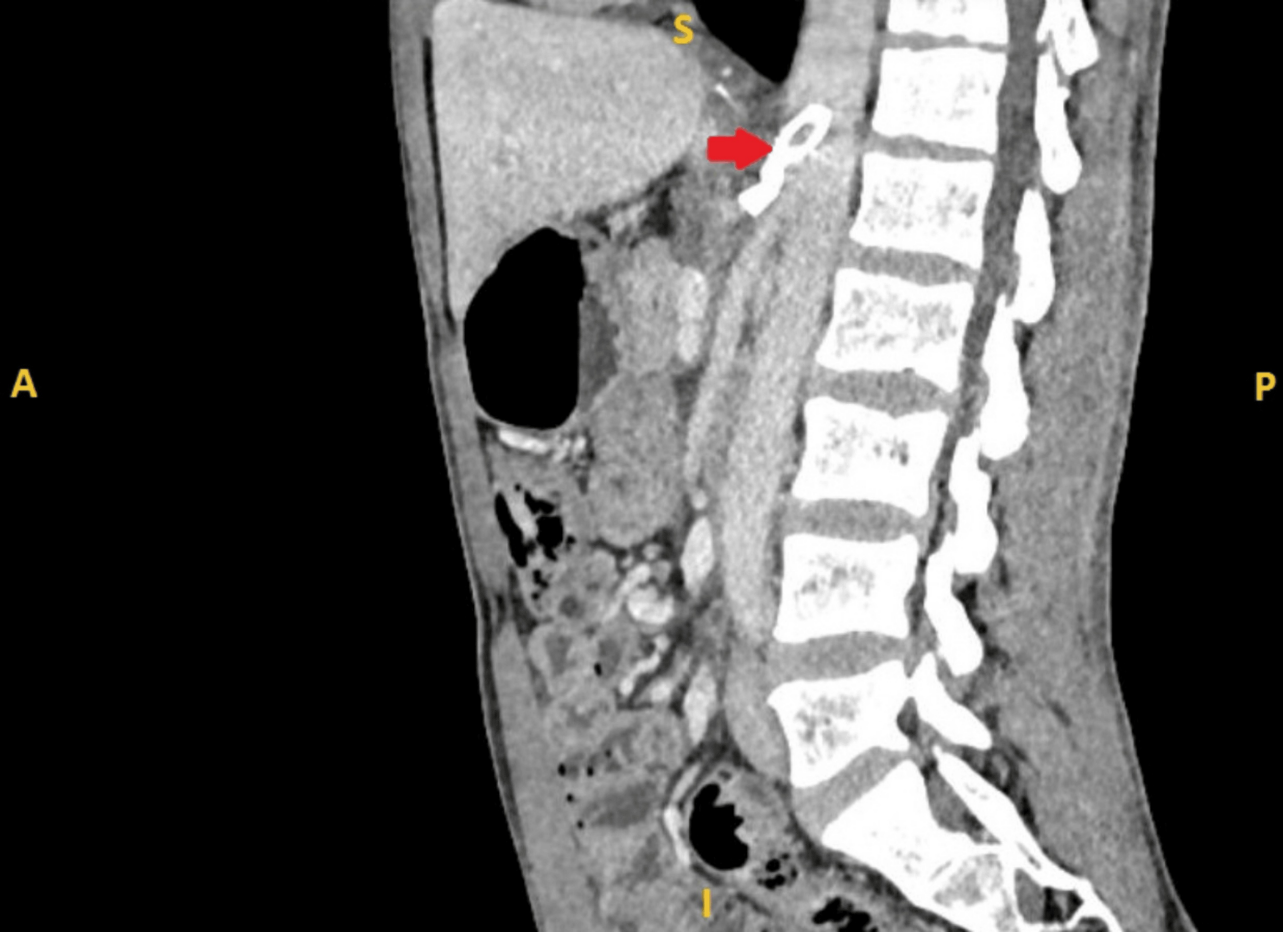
Postoperative contrast-enhanced CT scan of the abdomen (sagittal view) following celiac artery stenting showing stent in situ. Red arrow: celiac artery with stent in situ
